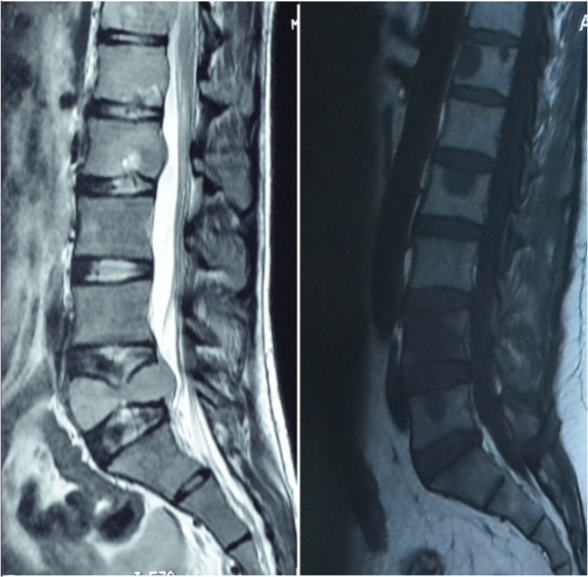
Spinal preoperative T1-weighted and T2-weighted magnetic resonance imaging revealed multilevel vertebral body edema
